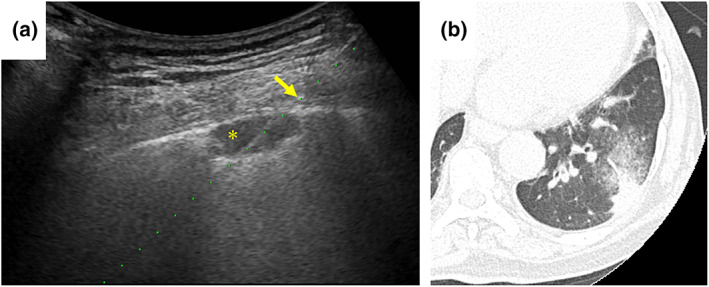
(a) Echo‐guided percutaneous needle biopsy was performed. 18‐G needle (arrow). A subpleural hypoechoic mass (asterisk). (b) After the biopsy, computed tomography (CT) shows no pneumothorax, hemothorax, or air emboli, but a localized ground‐glass opacity is seen around the mass.
